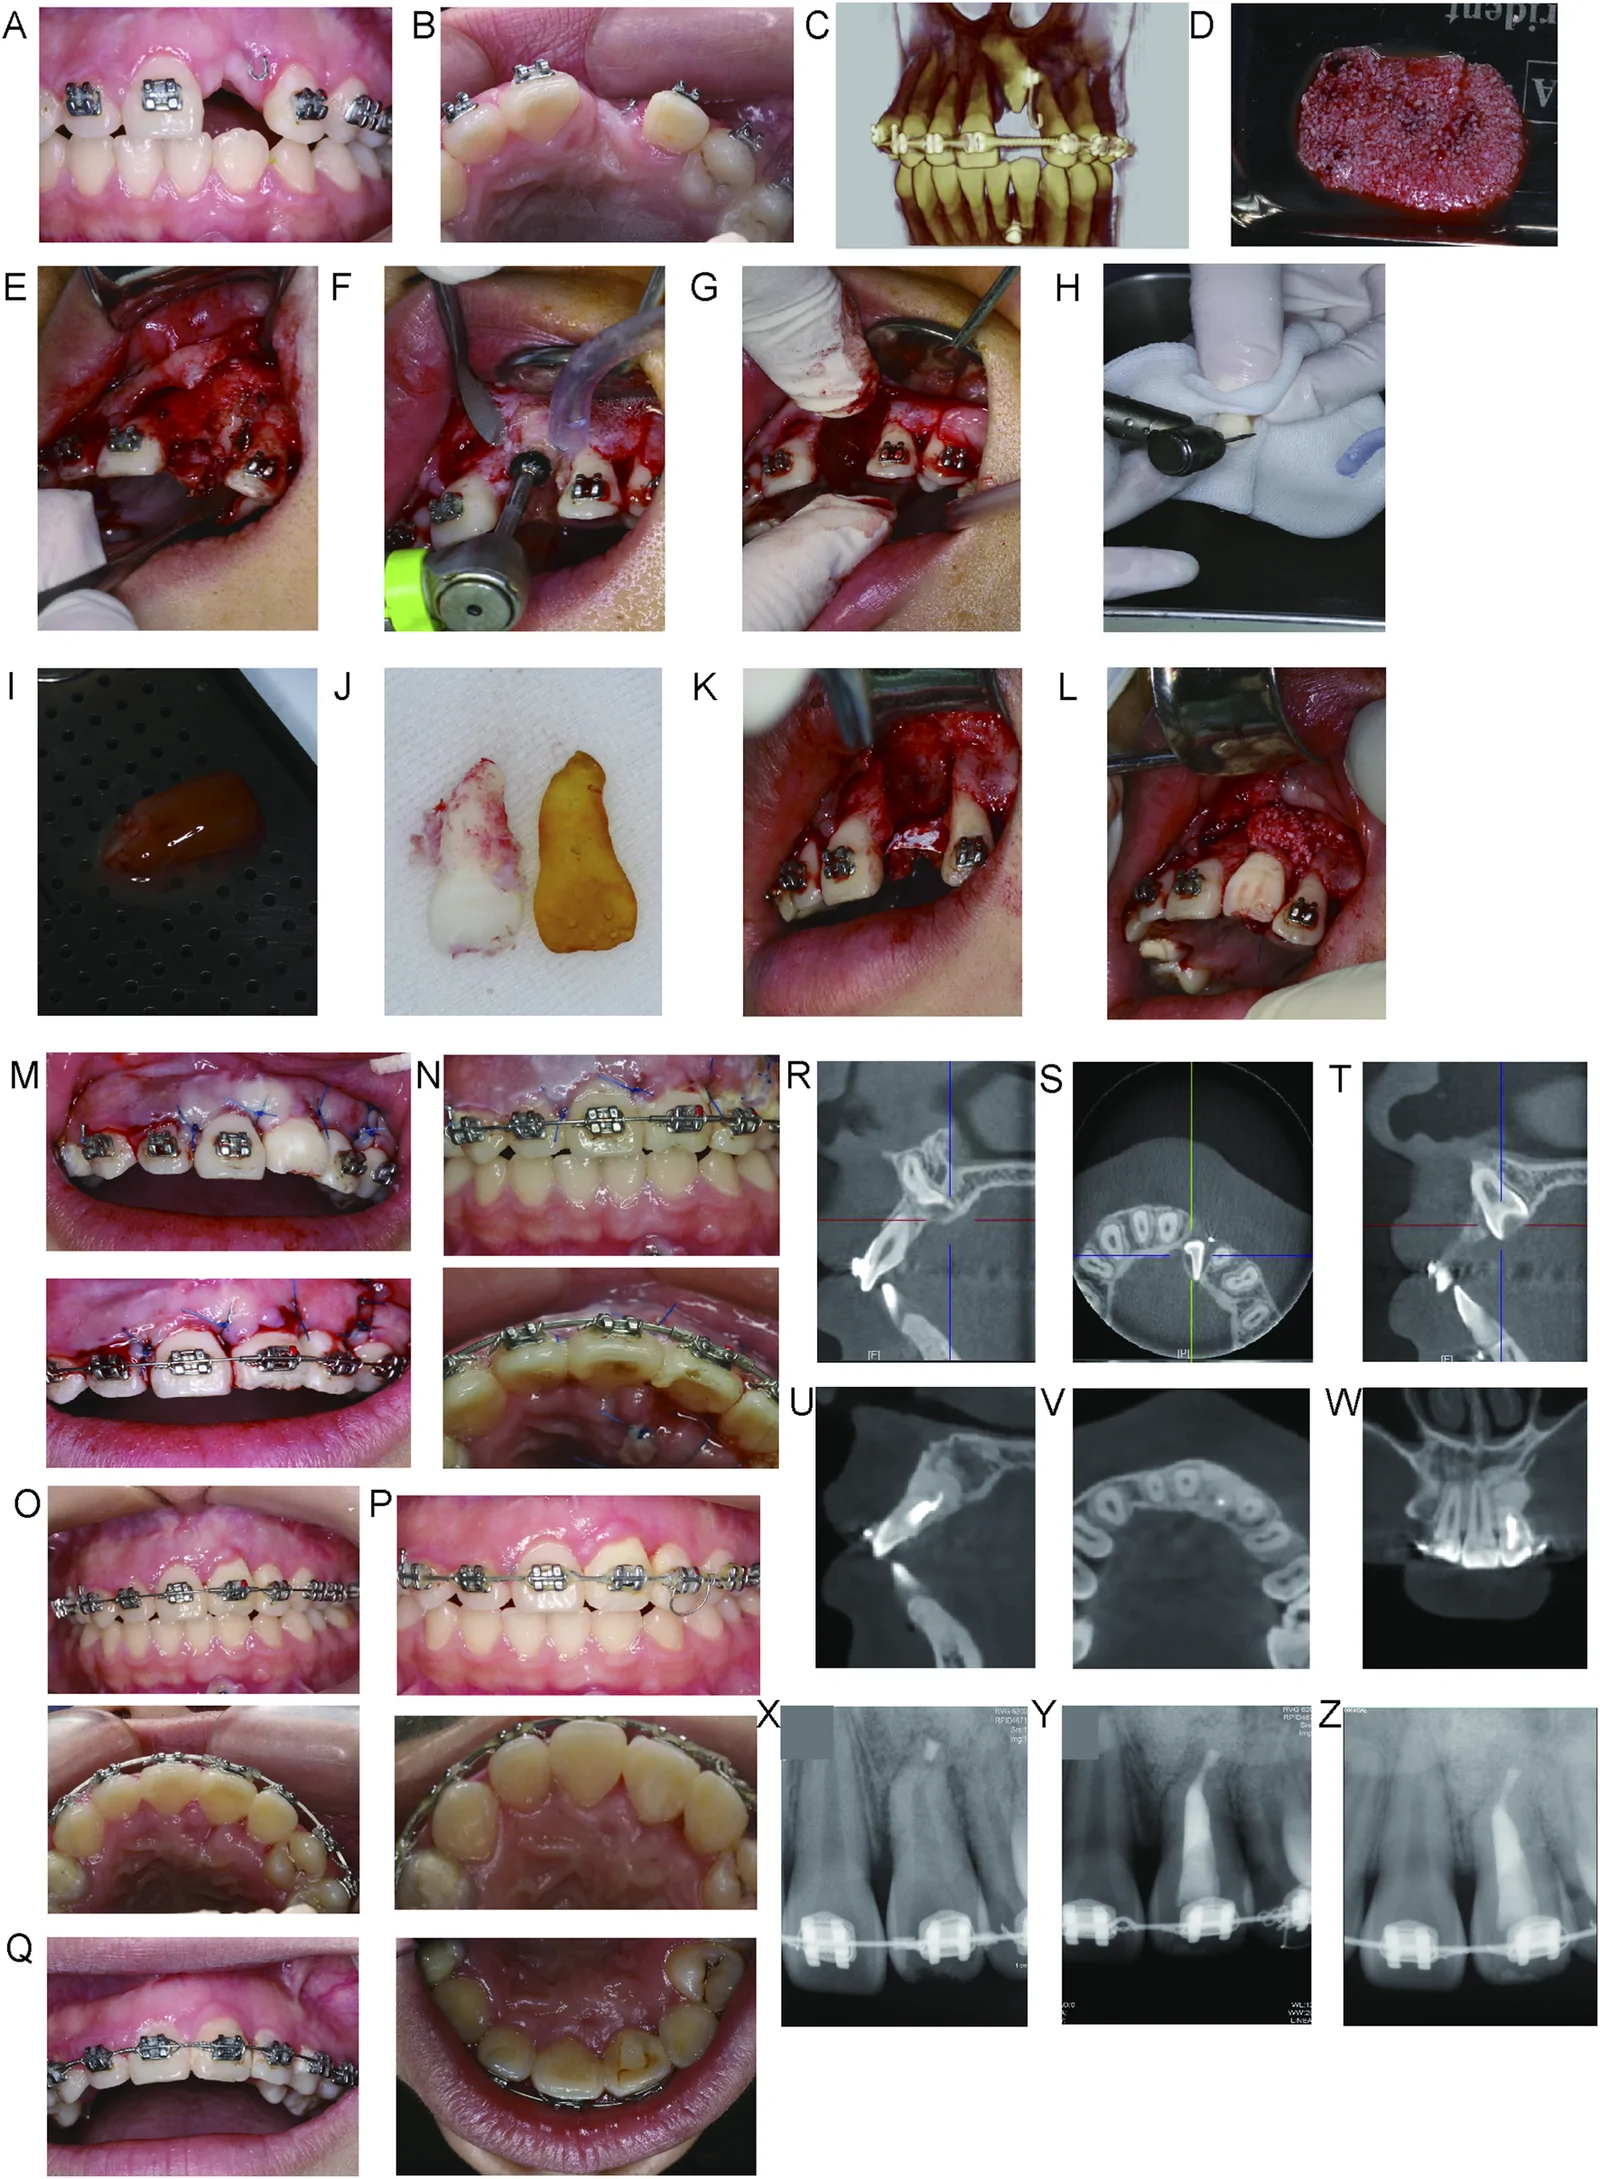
Clinical photographs and radiographic images of Case 4 showing digitally assisted autogenous transplantation of impacted tooth 21 to the maxillary anterior region. (A–C) Initial intraoral photographs. (D) Preparation of sticky bone. (E) Elevation of the labial mucoperiosteal flap and exposure of the recipient site. (F, G) Atraumatic extraction of impacted tooth 21. (H) Deliberate reduction of the interfering root prominence, root-end preparation, and retrograde filling. (I) Preparation of concentrated growth factor. (J) Comparison of the 3D-printed donor-tooth replica with the extracted tooth. (K) Placement of the resorbable collagen membrane. (L) Placement of sticky bone and transplantation of tooth 21 into the prepared recipient site. (M) Immediate postoperative intraoral photograph. (N) Intraoral photograph at 4 days. (O) Intraoral photograph at 7 days. (P) Intraoral photograph at 5 months. (Q) Intraoral photograph at 30 months. (R–T) Preoperative CBCT images. (U–W) CBCT images at the 30-month follow-up. (X) Immediate postoperative periapical radiograph. (Y) Periapical radiograph at the 5-month follow-up. (Z) Periapical radiograph at the 17-month follow-up. ATT, autogenous tooth transplantation; CBCT, cone-beam computed tomography; CGF, concentrated growth factor; 3D, three-dimensional.
